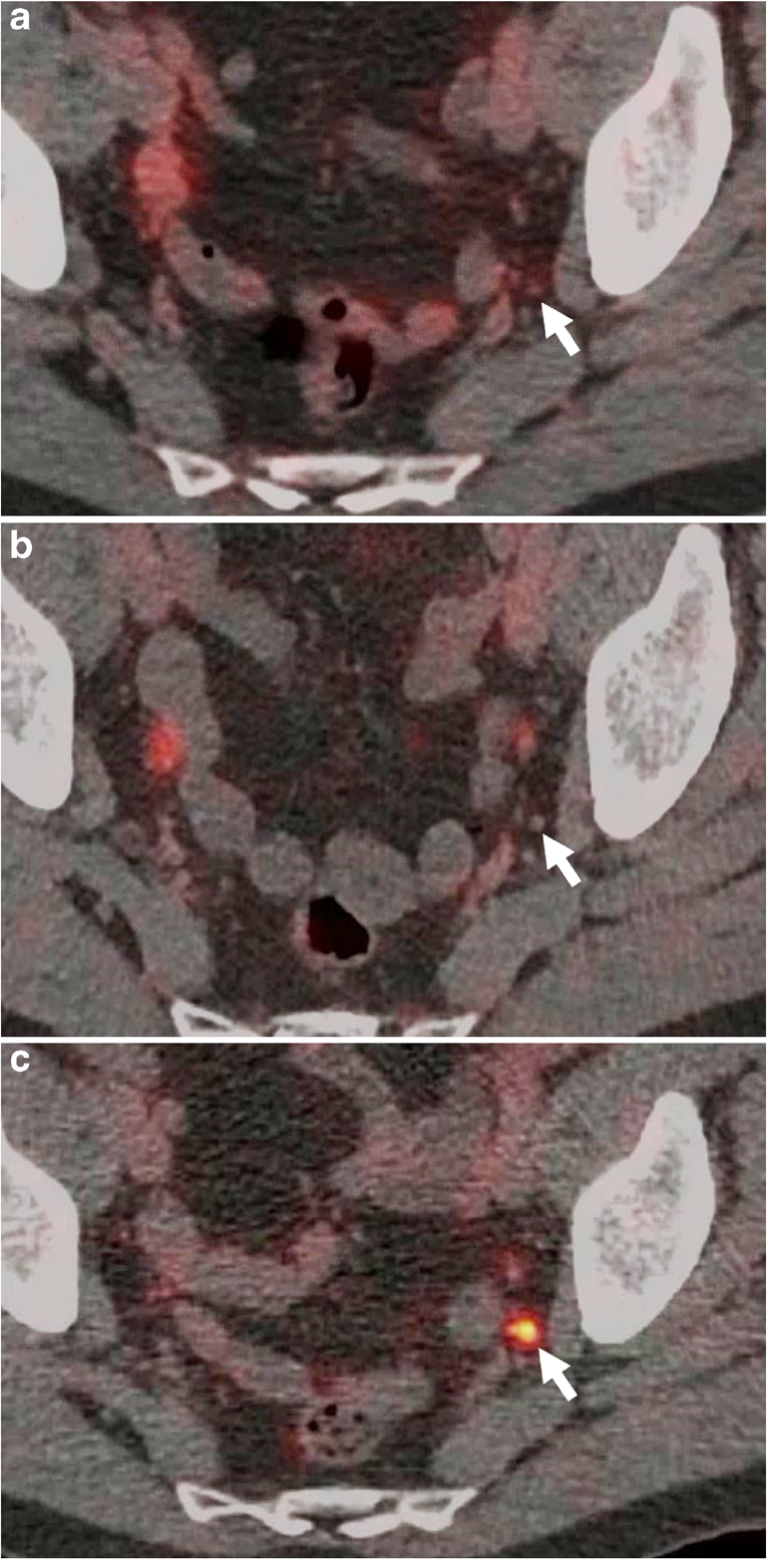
Serial Ga-68-PSMA-PET/CT in a 67-year-old man with BCR following radical prostatectomy and prostatectomy bed radiotherapy. a Initial scan at PSA 0.1 ng/ml. Left internal iliac lymph node (*arrow*) considered benign, morphologically (< 4 mm diameter) and metabolically (SUV 1.2). b PSA rise to 0.2 ng/ml, follow-up scan after 4 months. Minimal enlargement of the lymph node, still within normal limits (SUV 0.7; PSA). c Further PSA rise to 0.4 ng/ml, follow up scan 5 months after *b*. Lymph node remains subcentimetre (6 mm), but due to high PSMA uptake (SUVmax 8.2), now considered suspicious for malignant metastasis
